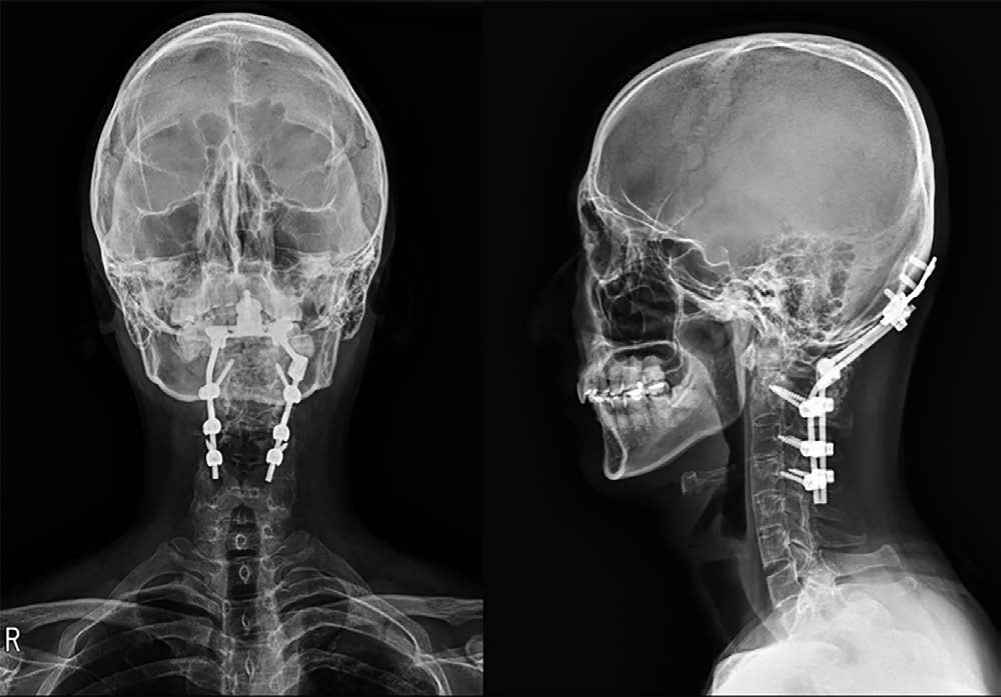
Post-operative X-ray showing a restoration of C1-C2 articulation and a reduction of the odontoid fracture.
